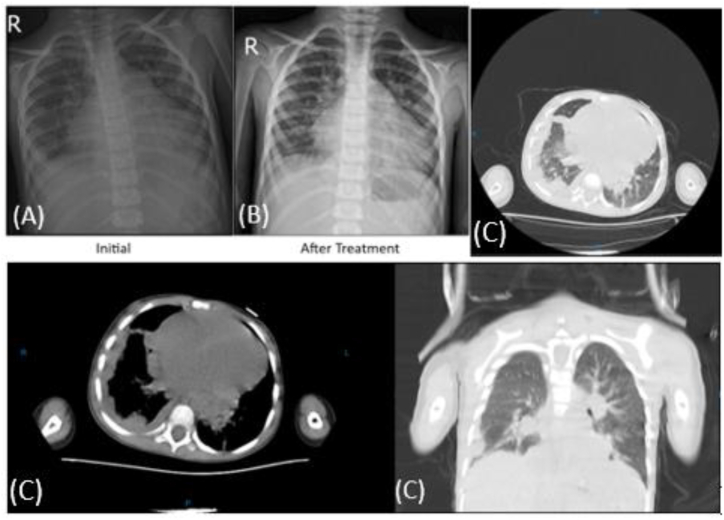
Radiologic findings of the patient. (A) Initial AP chest radiograph demonstrating cardiomegaly with diffuse bilateral interstitial and alveolar opacities, more prominent in the right lung. (B) Follow-up PA chest radiograph after 3 months showing partial resolution of pulmonary infiltrates. (C) Chest CT demonstrating right-sided loculated pleural effusion with pleural thickening, atelectasis, and multifocal right lung consolidation.
